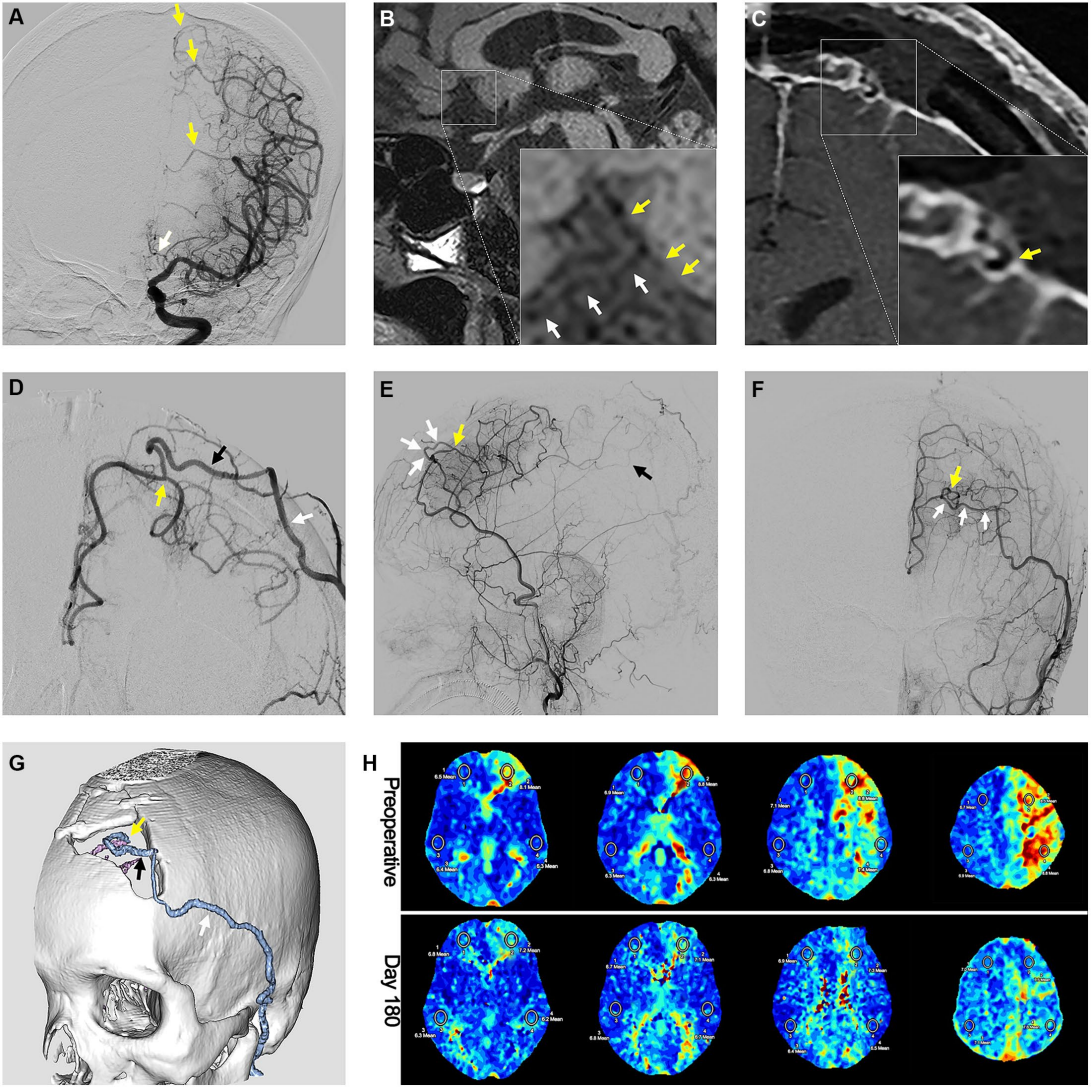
Preoperative and postoperative radiological images showing the efficacy of the parietal branch of superficial temporal artery-interposed superficial temporal artery-to-anterior cerebral artery bypass. (A) Preoperative DSA image showing the occlusion of the ACA. The yellow arrows indicate the compensation flow from cortical branches of the middle cerebral artery. The white arrow indicates the occlusion of ACA. (B) High-resolution MRI image showing atherosclerotic occlusion of the A2 segment. The yellow arrows indicate the atherosclerotic occlusion. The white arrows indicate the orbitofrontal branch. Postoperative (C) high-resolution MRI image and (D) DSA image showing the anastomosis of the pSTA and ACA. The yellow arrow indicates the anastomosis. The black arrow indicates the pSTA graft. The white arrow indicates the fSTA. Postoperative DSA images in (E) A-P view and (F) lateral view showing the ACA blood flow brought by bypass graft. The yellow arrow indicates the anastomosis. The white arrows indicate the pSTA graft. The black arrow indicates the inferior branch of internal parietal branches. (G) Computed tomography angiography showing the course of the pSTA graft. The yellow arrow indicates the anastomosis. The black arrow indicates the pSTA graft. The white arrow indicates the fSTA. (H) Perfusion improvement in the frontal lobe displayed by the mean transit time sequence of MRI. DSA, digital subtract angiography; ACA, anterior cerebral artery; MRI, magnetic resonance imaging; pSTA, parietal branch of superficial temporal artery. fSTA, frontal branch of superficial temporal artery.
